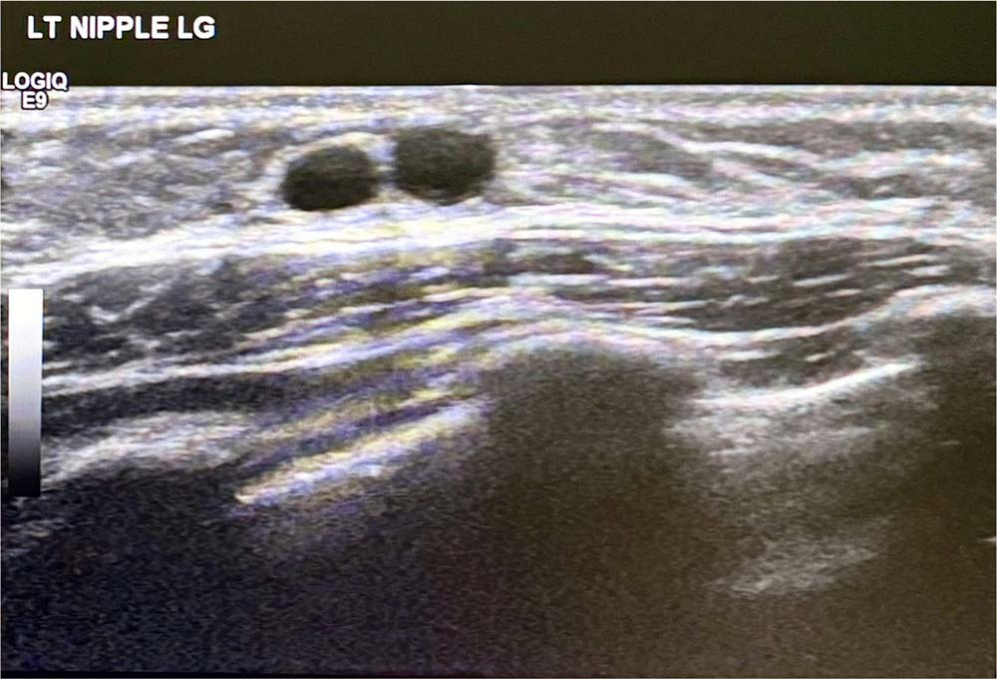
Ultrasound scan left breast showing cystic duct changes suggestive of mammary duct ectasia.
